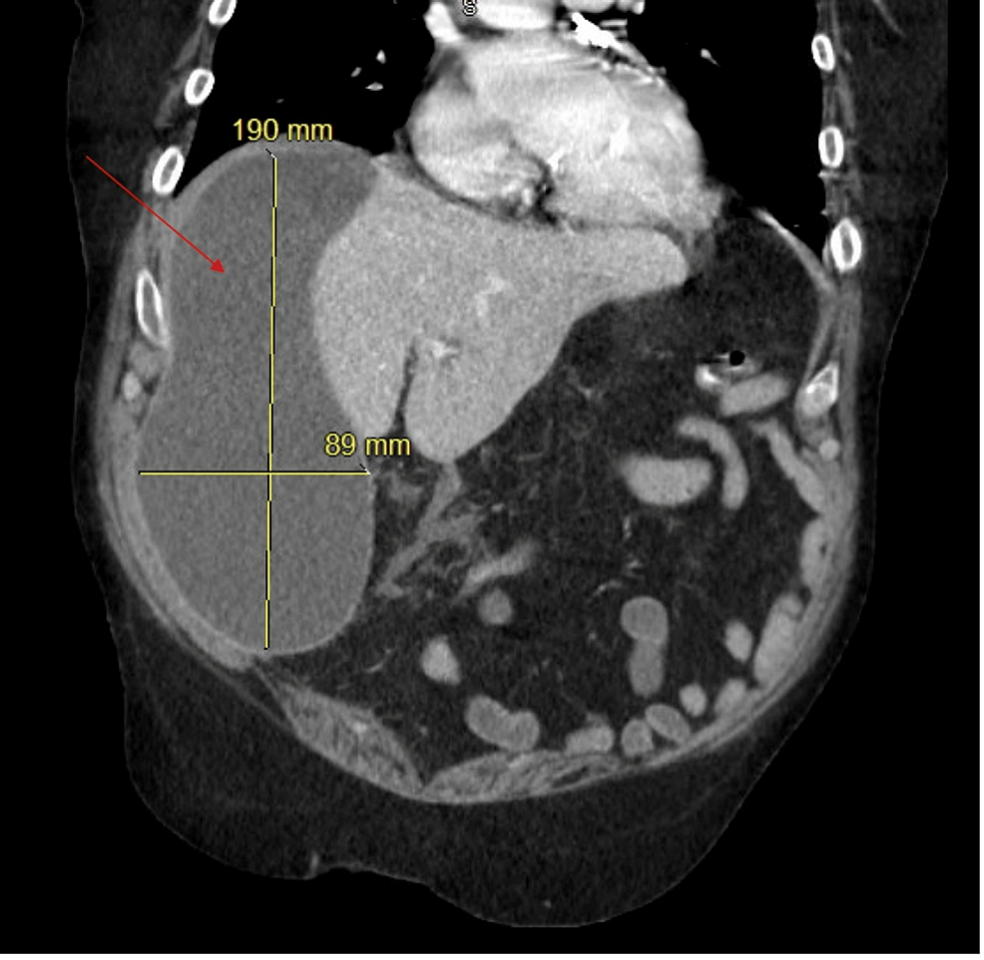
Coronal CT of the abdomen with subcapsular hepatic biloma measuring 19 x 8.9 cm. CT: computed tomography
